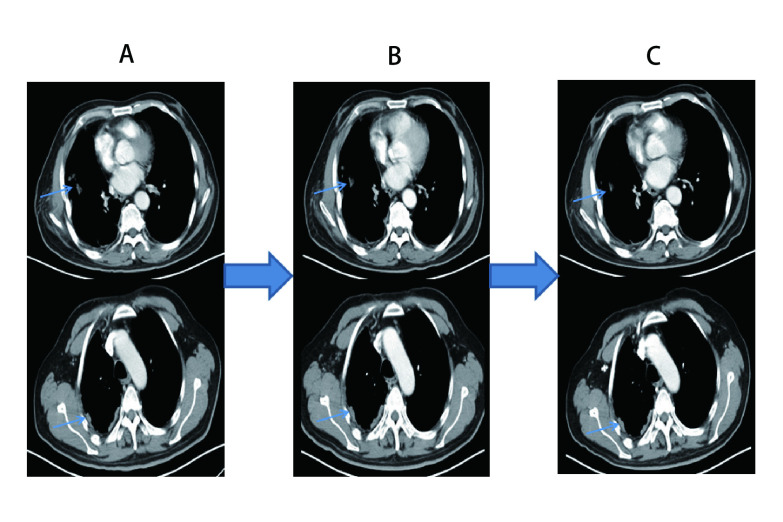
Nivolumab治疗前后的胸部CT表现。A：应用Nivolumab治疗前，胸部CT显示右肺、右侧胸膜多发转移；B：应用3次Nivolumab治疗后胸部CT显示转移灶稳定；C：停用Nivolumab免疫治疗7周，胸部CT显示疾病稳定，右肺、右侧胸膜转移灶较前稳定略缩小。 Chest CT scans before and after Nivolumab. A: The chest CT scans before Nivolumab at different layers showed multiple metastases in right lung and pleura; B: The chest CT scans after 3 doses of Nivolumab treatment revealed the metastases remained stable; C: After discontinuation of Nivolumab treatment for 7 weeks, the chest CT scans showed that the metastatic nodules were stable and slightly smaller than those before.
